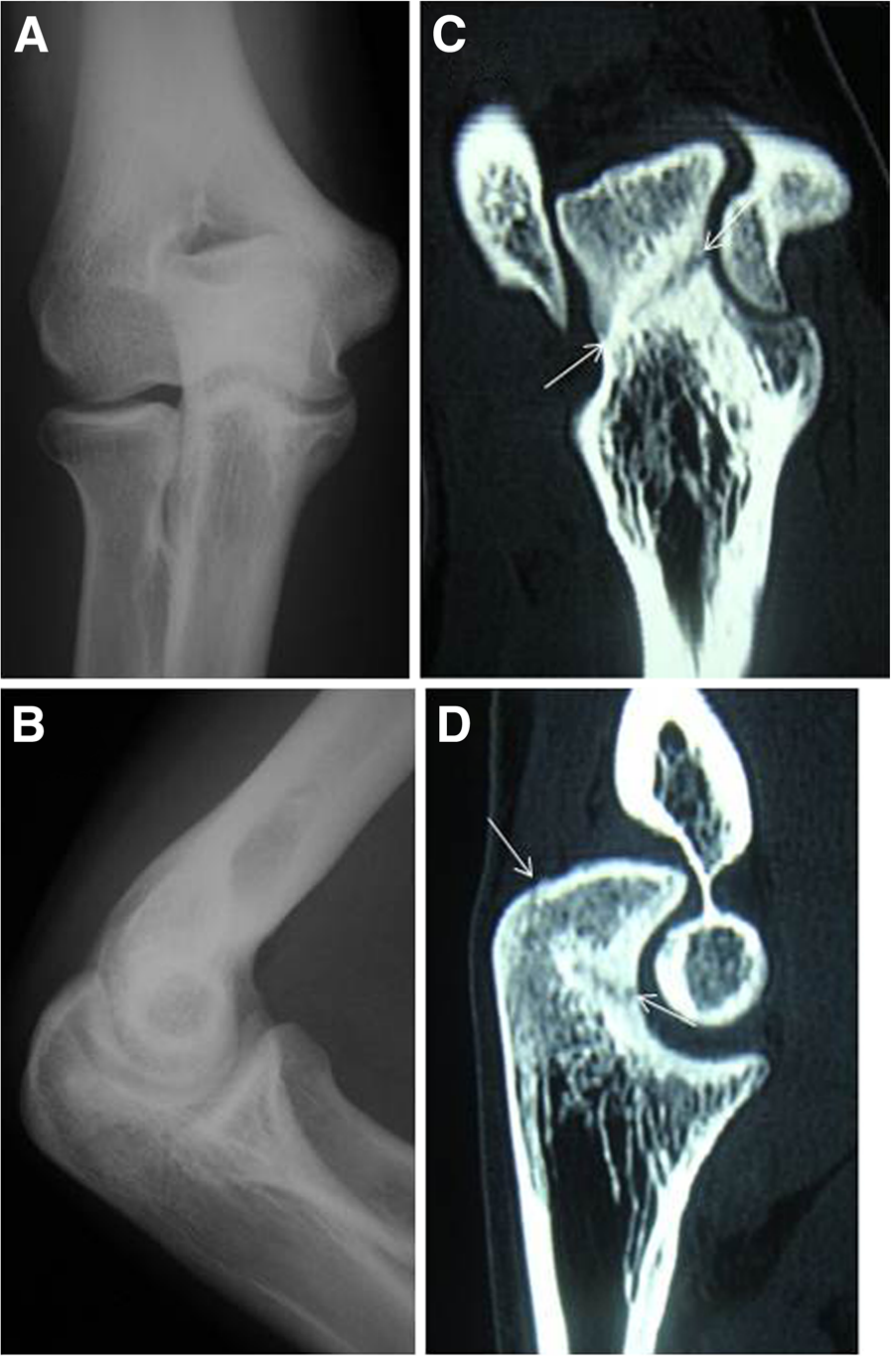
Case 2. Radiographs and CT scan of the elbow before surgery. There were no significant signs such as fracture or osteoarthritic change on anteroposterior (A) and lateral (B) views of radiographs. On the frontal (C) and sagittal plane (D) of CT scan, non-displaced and straight oblique fracture line (arrow), from medial proximal to lateral distal at the middle third of the olecranon, was detected.
